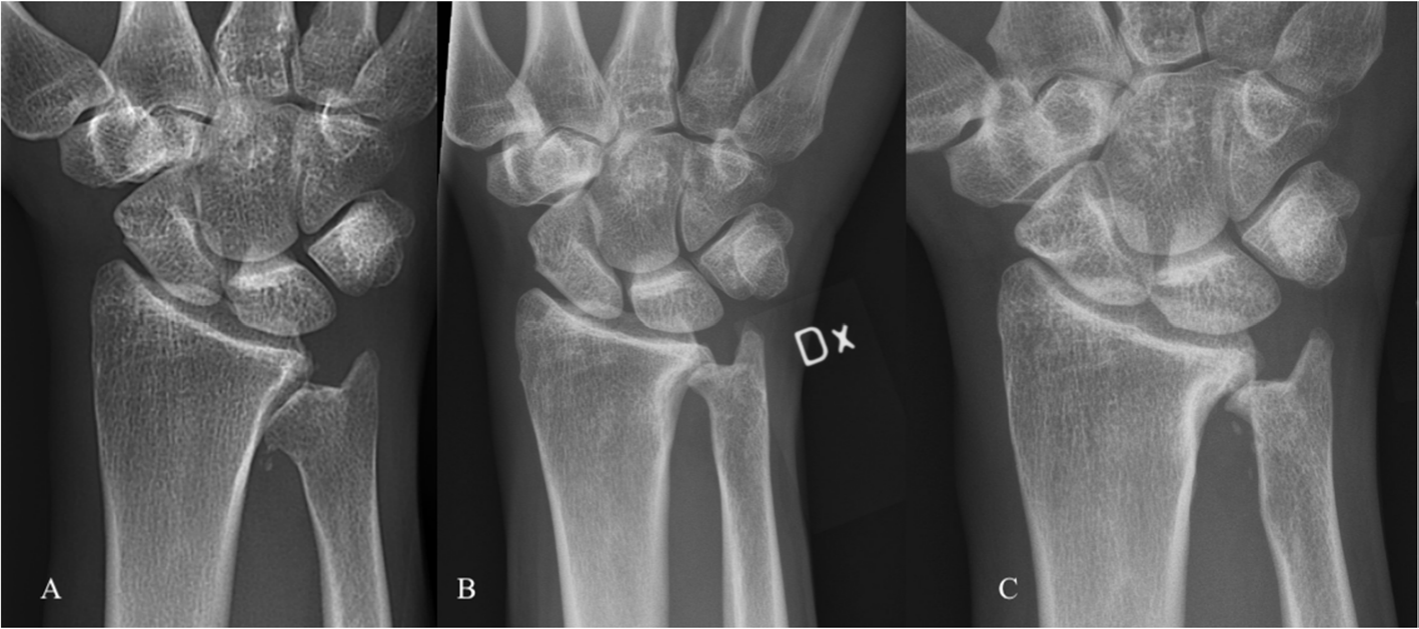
Posterior-anterior (PA) view with conventional radiography (CR) in case 1 with preoperative signs of osteoarthritis in the DRUJ (A). An increased distance in the DRUJ at 2.5 years follow-up (B), and similar findings 5 years postoperatively (C)
